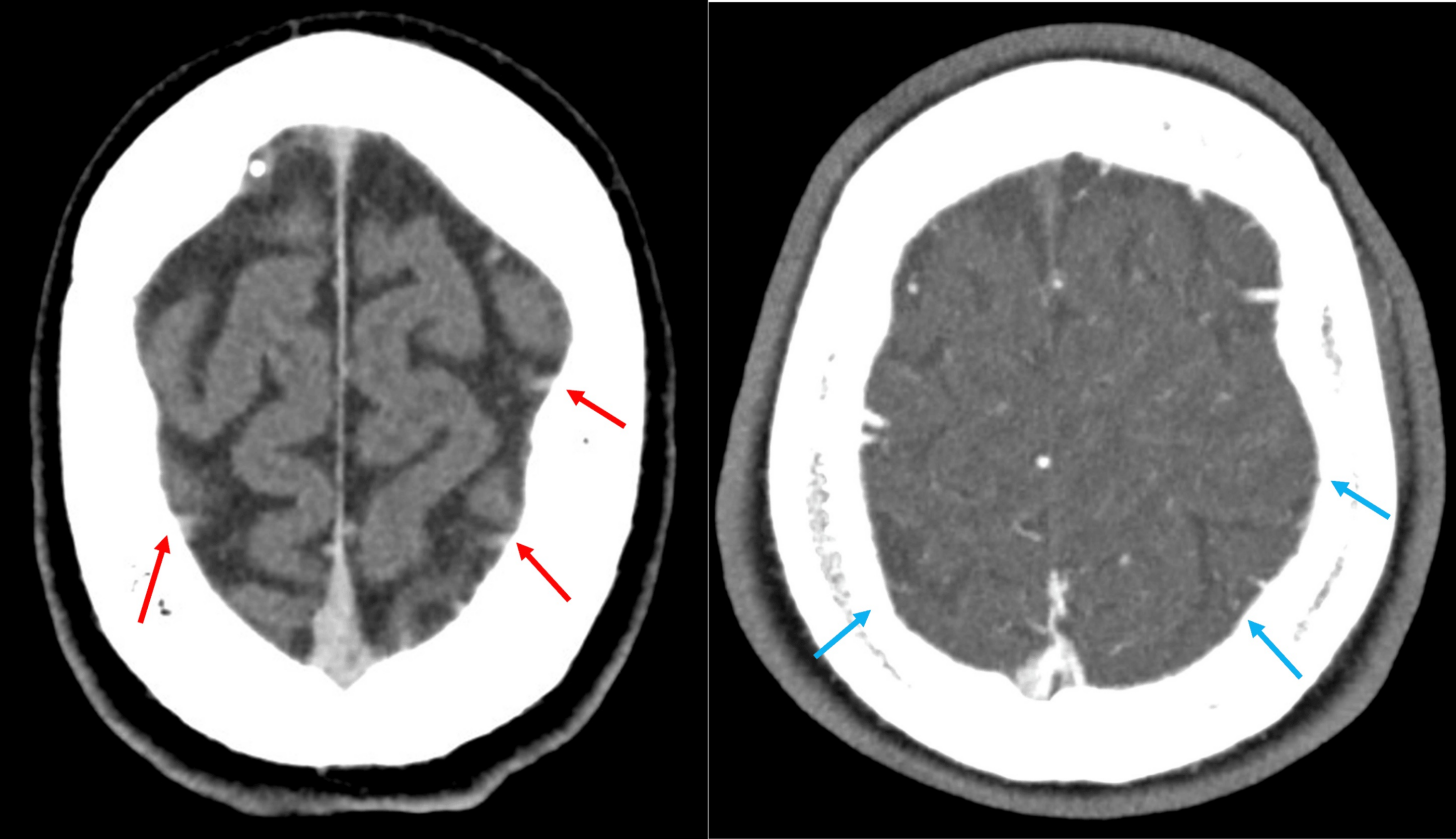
Initial CT brain (15/09/24) image reported showing no acute abnormalities. The red arrows indicate areas of hyperdensity that, in retrospect, could represent thrombi on a non-contrast scan. The blue arrows indicate areas where there is no flow on the CT angiogram, demonstrated by a lack of hyperdense white contrast, further indicating the presence of a thrombus. Although not a dedicated venogram, there is a filling of venous sinuses visible superior to the blue arrows.
